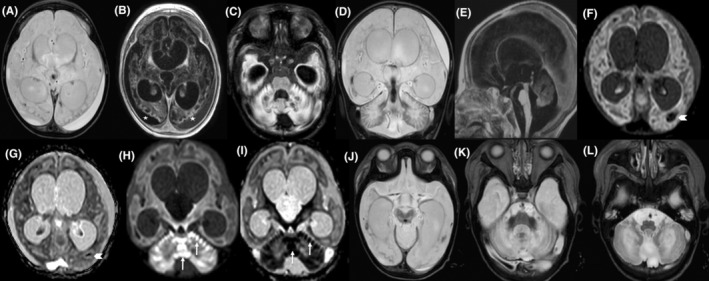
Brain MRI of a 16‐month old boy (case 1) with mutation in *NARS2*. Axial T2‐Weighted, T1‐Weighted and FLAIR sequences, respectively (A–C), and coronal T2‐Weighted image (D) show significant supra and infratentorial parenchymal destruction as white matter cystic change and vacuolization with involvement of U fibers, involvement of cerebral and cerebellar cortex, abnormal T2 hyperintensity and T1 hypointensity, cystic change and atrophy of basal ganglia and thalami, involvement of dentate nuclei and ventriculomegaly. The cerebral atrophy and secondary subdural hematoma in different ages are shown by white stars‐B. In sagittal T1‐Weighted image (E) note the cystic change and abnormal signal intensity of vermis. Axial diffusion weighted images (DWI) images (F, H) and ADC map sequence (G, I) demonstrate parenchymal hemorrhage (white arrowheads) as blooming artifact and restriction of vermis and cerebellar cortex (white arrows) representing acute phase in restricted areas. Axial T2‐Weighted views (J–L) show mid brain, pons and medulla oblongata involvement, respectively.
